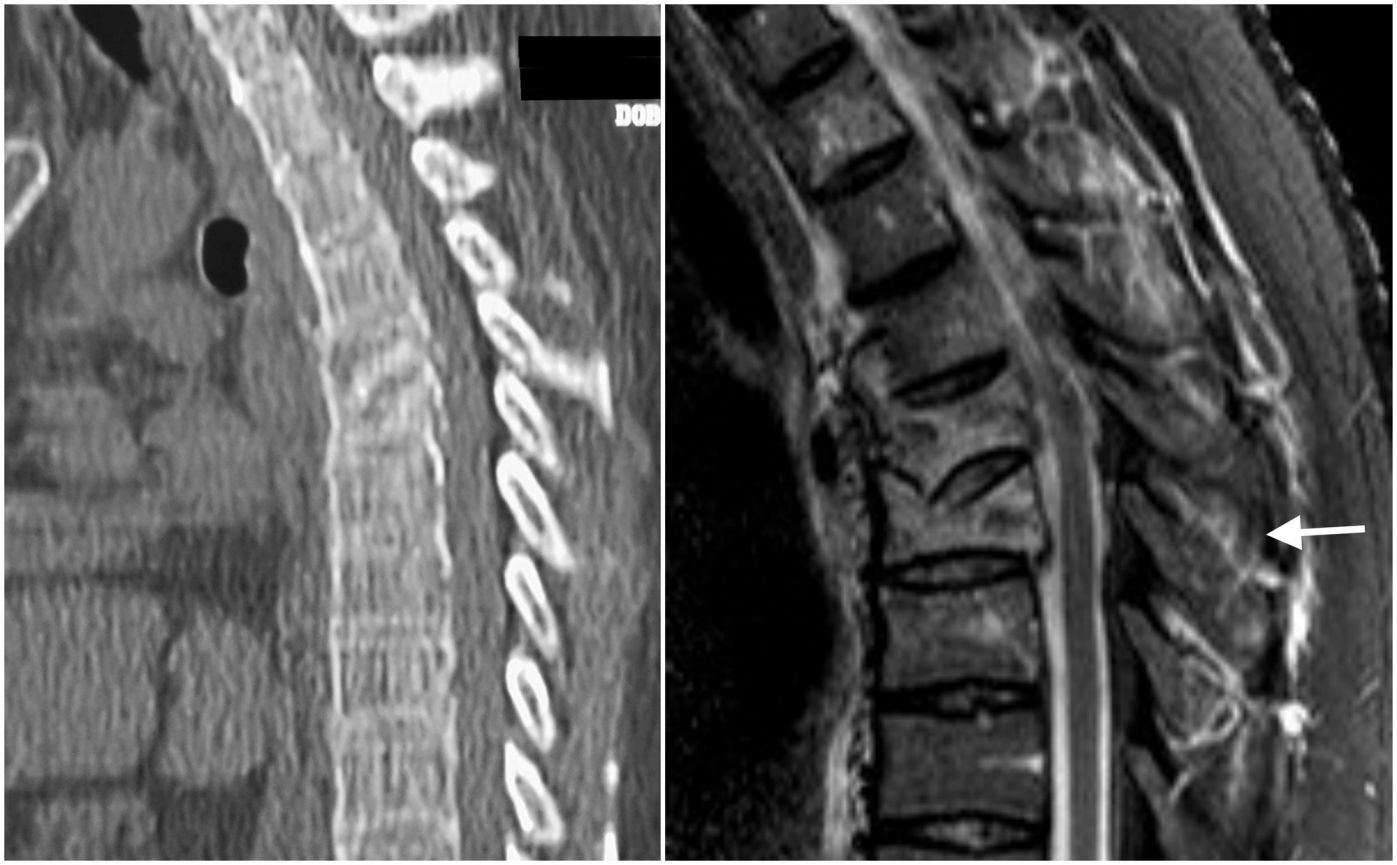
Thoracic fracture Left, sagittal computed tomography (CT) scan showing compression fracture of T4-5 vertebral bodies. Right, sagittal magnetic resonance imaging (MRI) STIR showing the fractures and changes in the intensity of vertebral bodies. In addition, injury to interspinous ligaments is observed (arrow). STIR - Short-TI Inversion Recovery.
